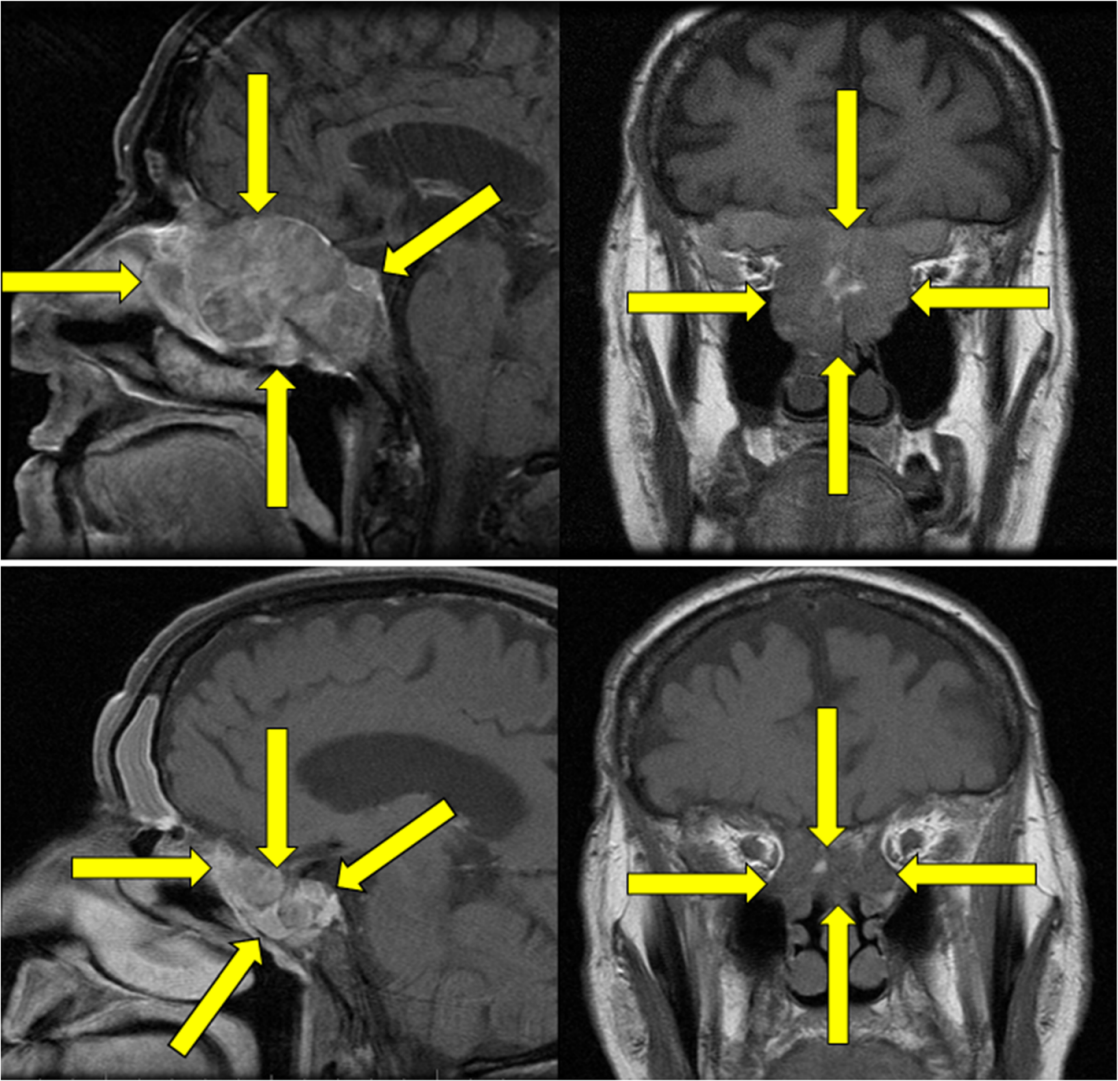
Baseline brain MRI images showing initial primary mucosal melanoma lesion. Primary melanoma centered in the sphenoethmoidal sinuses. Representative sagittal and coronal T1 images are displayed. The upper boxes correspond to the initial MRI, while the lower boxes reflect post-treatment images. Yellow arrows denote approximate tumor boundaries
